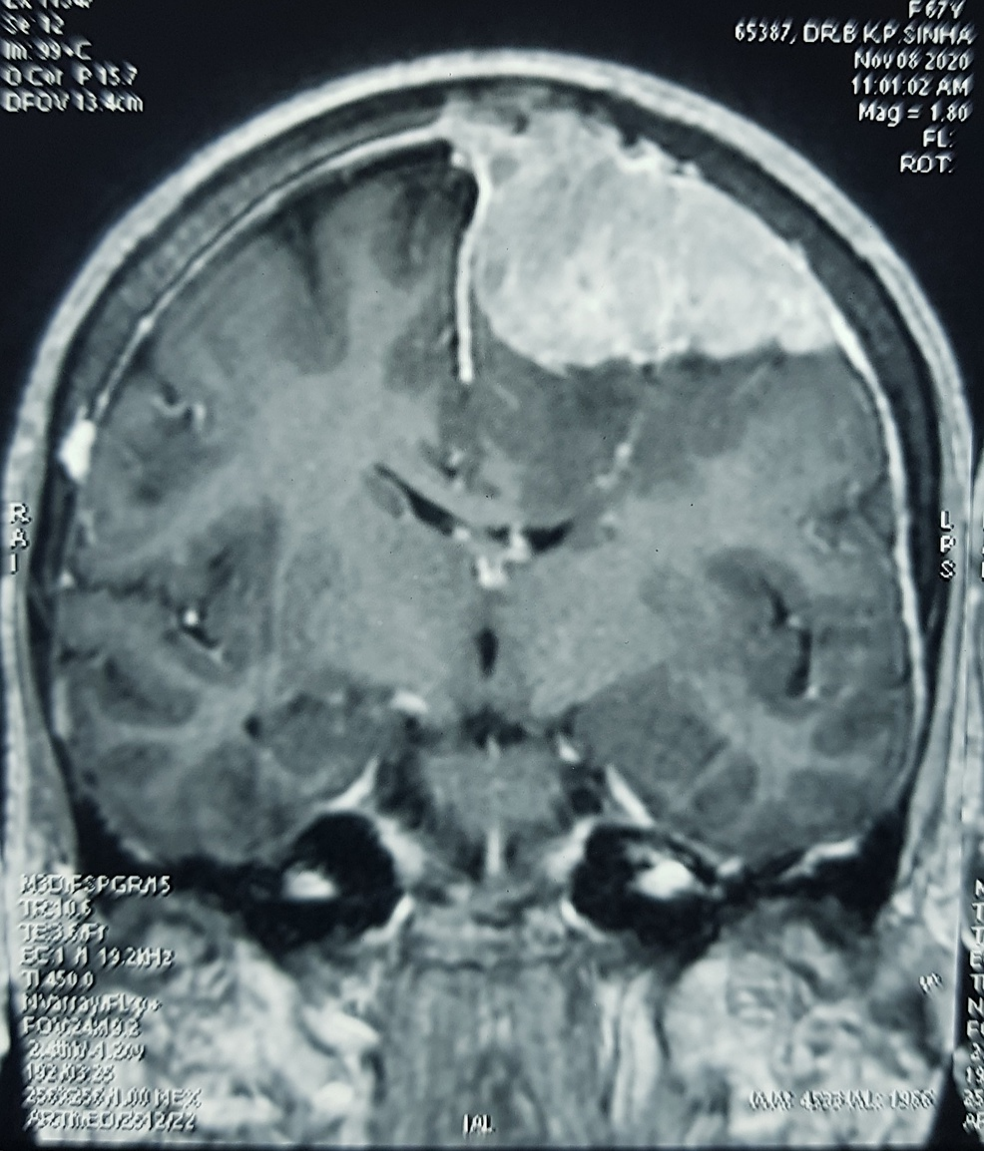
Pre-operative MRI coronal image.
